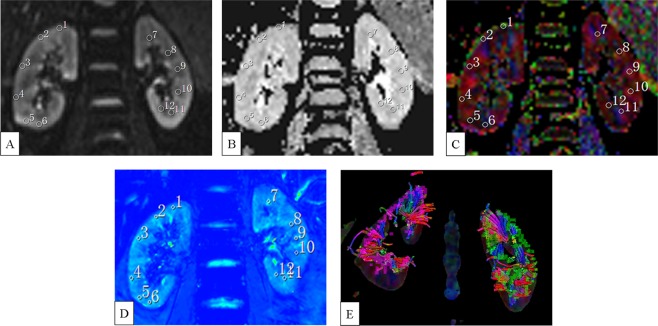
Coronal MR images of a 27-year old female. Six ROIs were drawn from upper to lower pole in the cortex and medulla respectively on DW (b = 0) image (A). The corresponding ROIs’ values in ADC map (B) and FA color map (C) were acquired automatically. Similarly, six ROIs were drawn from upper to lower pole in the cortex and medulla respectively on T2* map (D). The reconstructed FA Tractography of both kidneys (E) shows the orientation of medullary tubules. Note: Number 1 to 6 represent ROIs in the cortex, and number 7 to 12 in the medulla.
